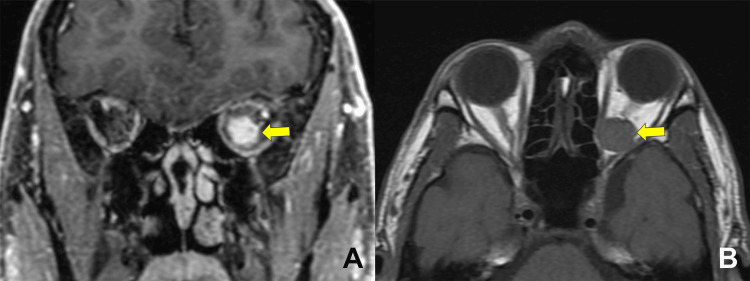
Preoperative magnetic resonance images. A T1-weighted enhanced coronal (A) and axial images (B) showing an orbital apex tumor (arrows) surrounded by the optic nerve and medial and inferior rectus muscles.
